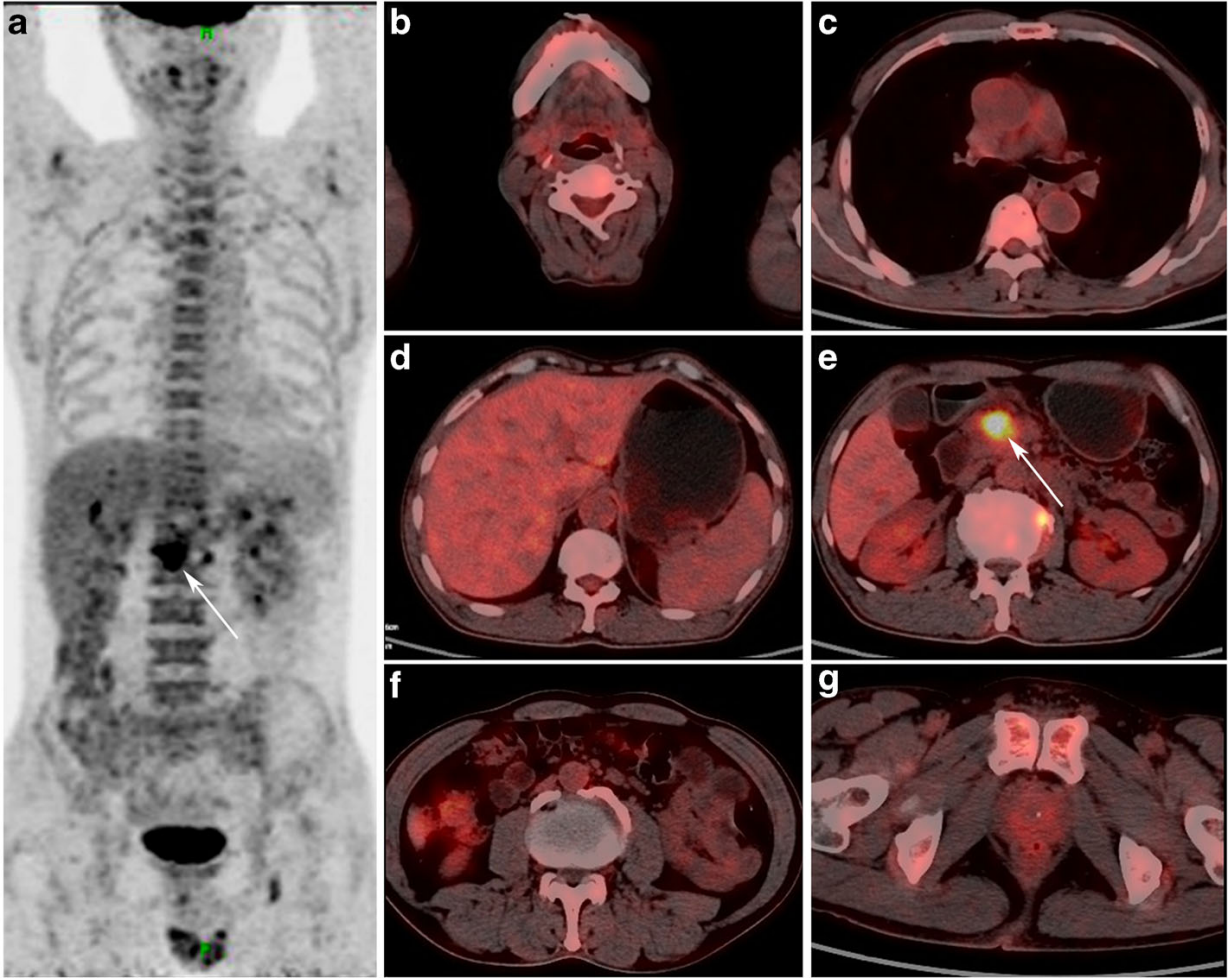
A 59-years old male patient with pancreatic cancer. MIP PET (a) and PET/CT fusion (e) images show a mass in the pancreatic head with increased FDG uptake (arrow), with early and delayed SUVmax of 9.3 and 10.8, respectively. Compared with AIP patients in Fig. 2, no increased FDG uptake foci in the salivary gland b), mediastinal lymph nodes (c), retroperitoneal space (f), and prostate (g) are observed, as well as no bile duct expansion (d)
